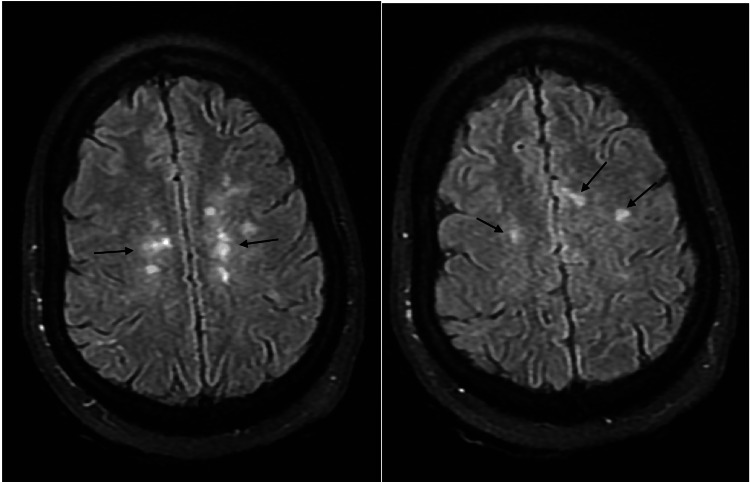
MRI brain T2 FLAIR showing multiple sub-cortical demyelinating lesions (black arrows) FLAIR: fluid-attenuated inversion recovery
